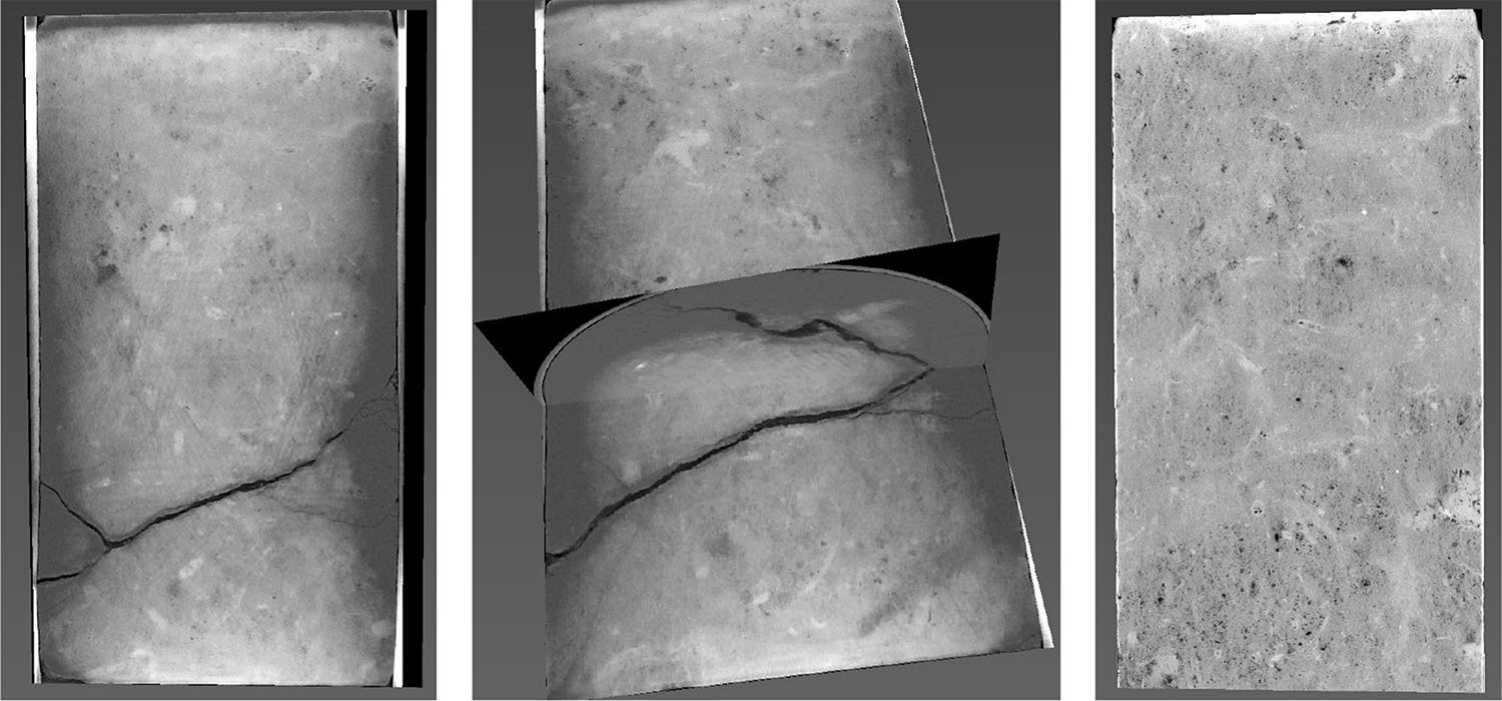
Vertical slices along the central axis of a 3D CT scan of C23 after the loading experiment shown at different image orientations (first two frames) and a pre-test vertical slice of a 3D CT scan of the same sample (third frame). The sample is 1 inch in diameter and 2 inches long.
